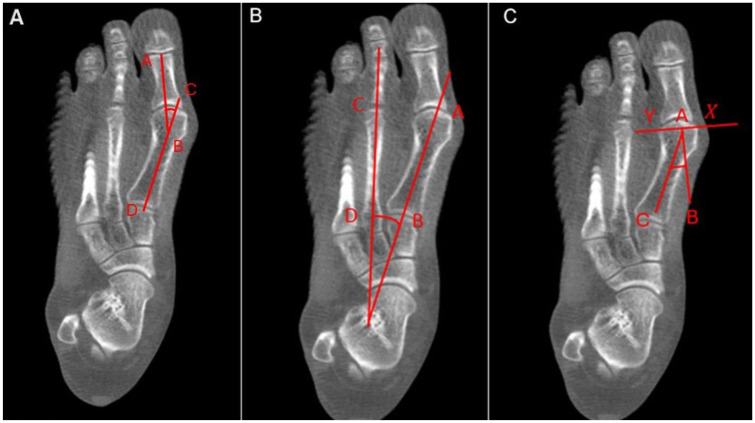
Panel A shows the measurement of the hallux valgus angle (HVA), and panel B shows the measurement of the 1-2 intermetatarsal angle (IMA). Panel C shows the measurement of the distal metatarsal articular angle (DMAA) on a digitally reconstructed radiograph.
